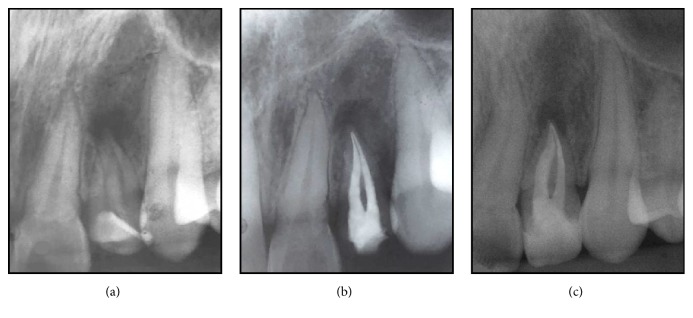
Operative imaging sequence. (a) Initial radiograph suggesting a geminated tooth; (b) final radiograph; (c) follow-up over 6 months' radiograph.
